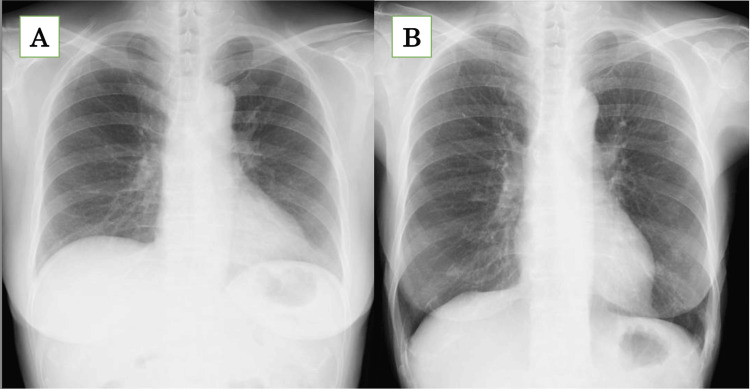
Changes over time in chest radiographs There are no abnormal findings in the lung fields, indicating that the lungs have become hyperinflated over the past four years. A: four years ago; B: just before the last recurrent malignant lymphoma
